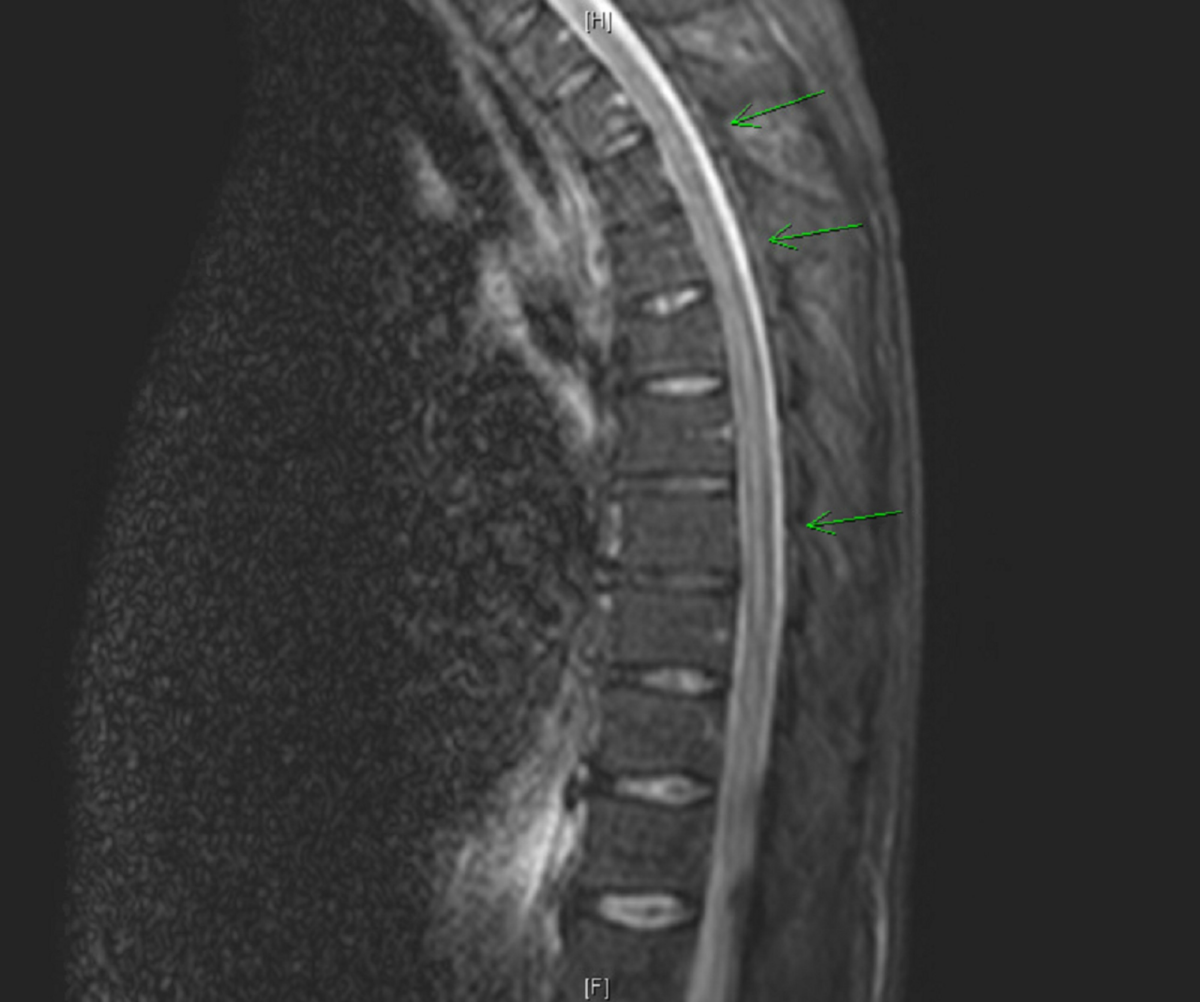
MRI demonstrating an abnormally increased intensity of the thoracic cord (green arrows)
